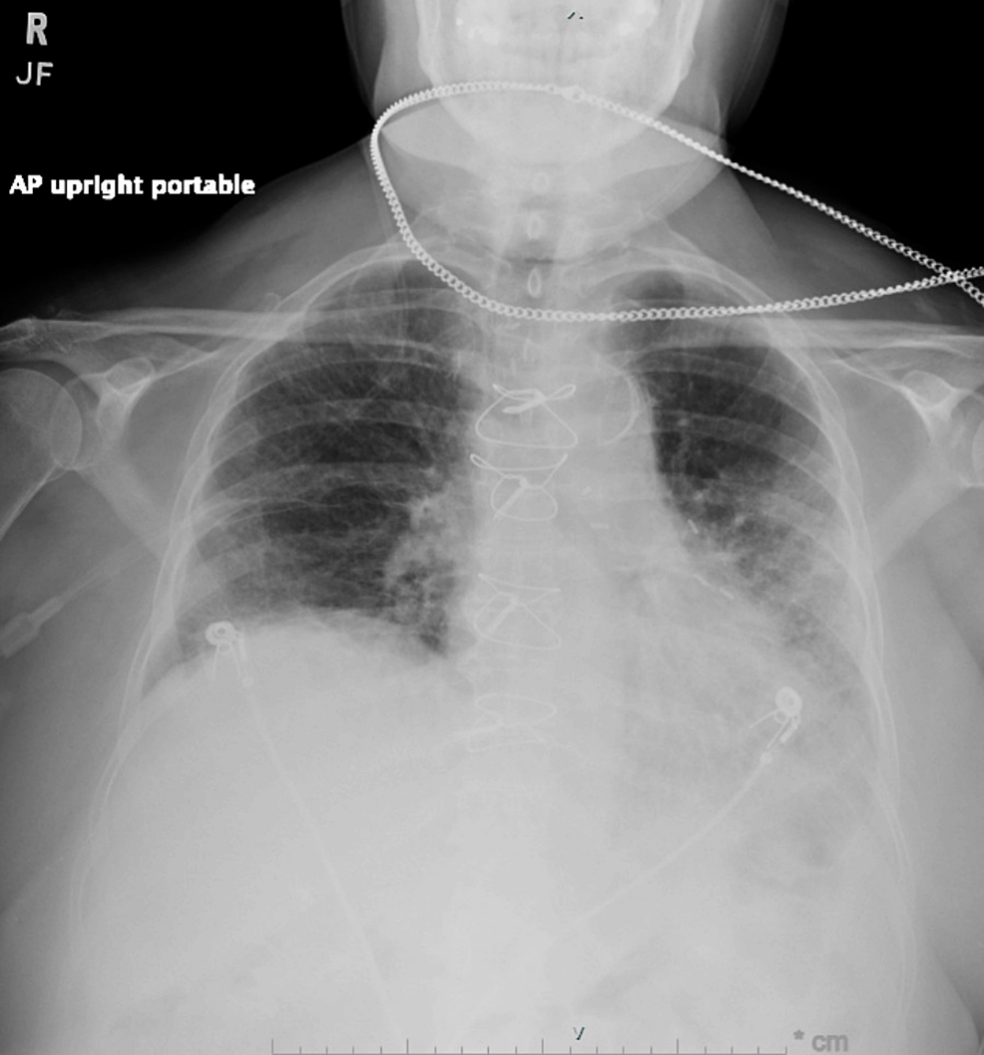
Chest x-ray The patient's chest x-ray revealed bilateral infiltrates with a small right pleural effusion
